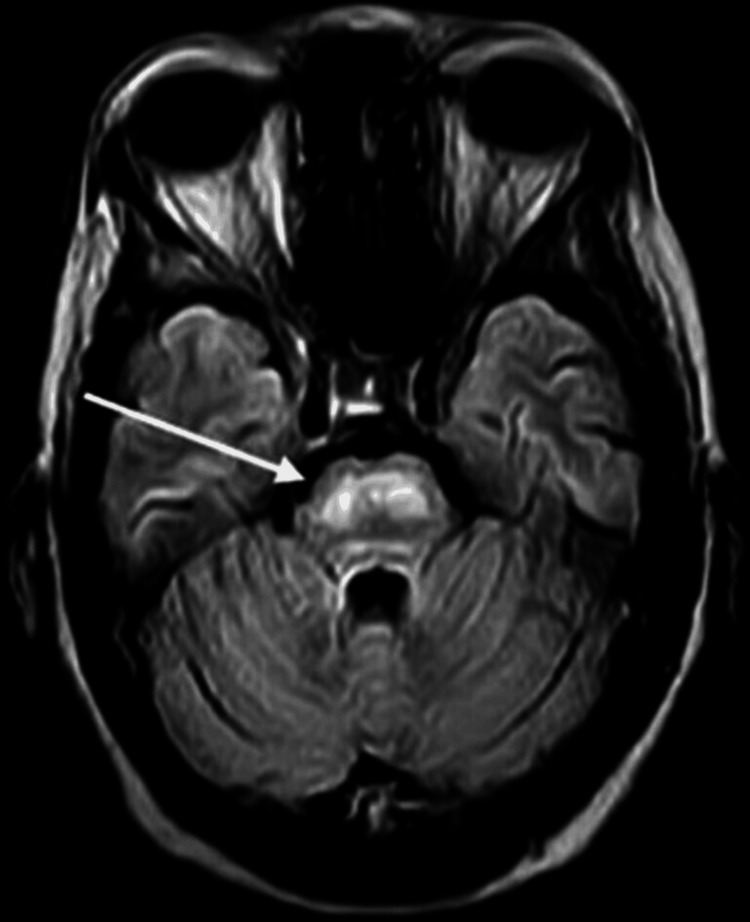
Persistence of abnormal T2/flair hyperintensity centrally in pons with peripheral sparing on magnetic resonance imaging.
